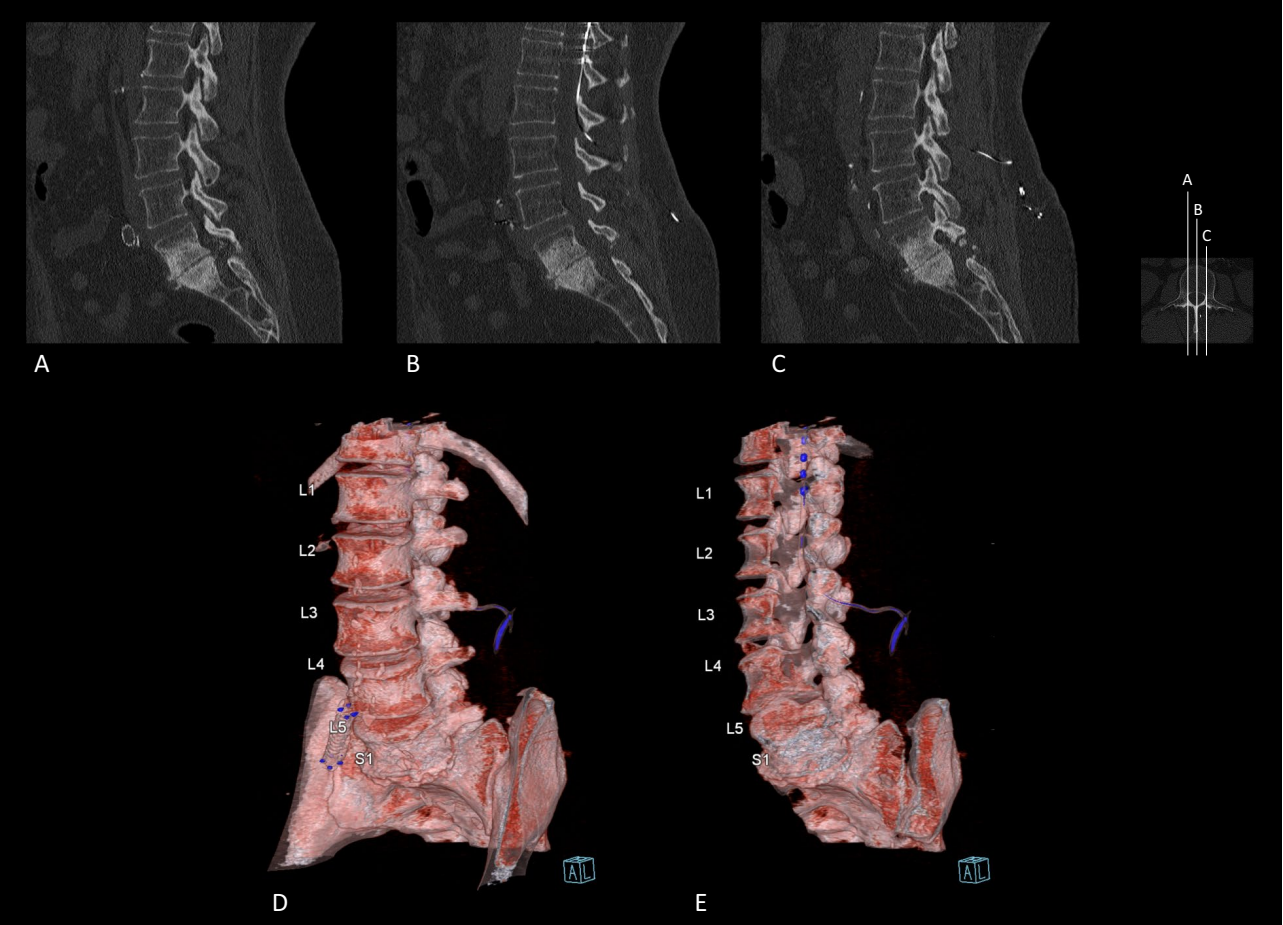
Lumbar spine CT images in an FBSS patient with an implanted spinal cord stimulation system. A–C Sagittal reconstructions through the vertebral column as depicted by the axial miniature on the right-hand side. The spinal cord stimulation electrode can be observed lining the ventral aspect of the dorsal lamina on level Th12–L1. Sclerosis of L5 and S1 can be observed. Additionally, at level L5–S1, bilateral laminectomy has been carried out. D Three-dimensional reconstructions of the spine of the same FBSS patient. The connection of the extension cables to the internalized pulse generator can be appreciated in blue at level L3–L4. E Virtually dissected three-dimensional model of the spine of the same FBSS patient. The spinal cord stimulation electrode can be appreciated as multiple blue dots in the spinal canal. The extension cable can be followed into the paraspinal space
